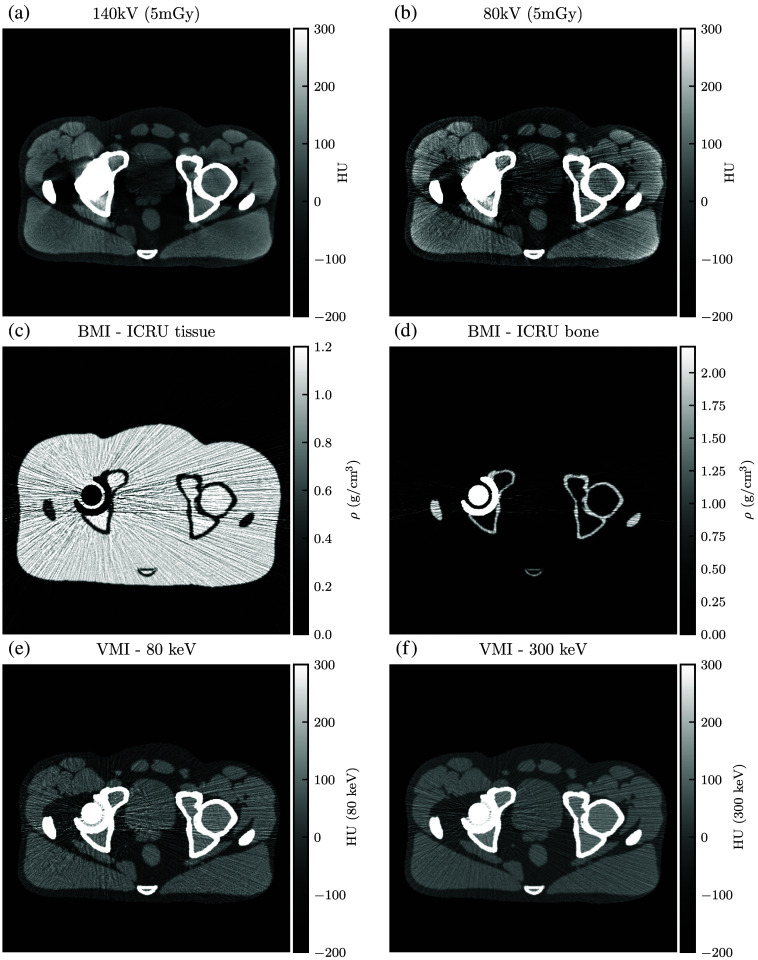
(a) and (b) Single-energy images, (c) and (d) BMIs, and (e) and (f) VMIs for the 140 kV-80 kV DE-CT acquisition with titanium hip replacement. In the VMIs, the beam hardening artifact of the single-energy images has been corrected, but there is residual streaking.
